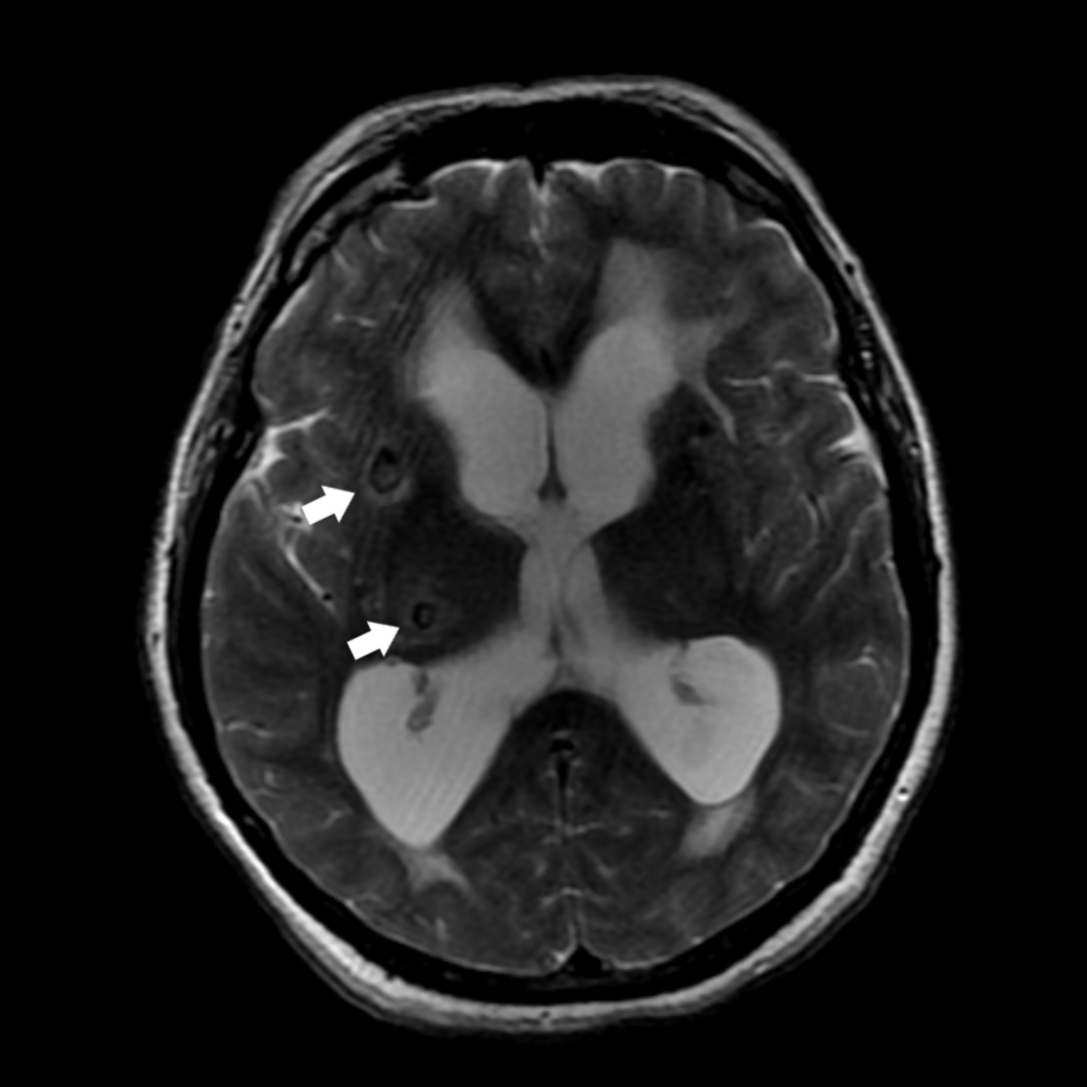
MRI T2-weighted axial. T2-weighted axial MRI image showing subcortical cystic lesions with perilesional hyperintensity and presence of scolex (arrows).
